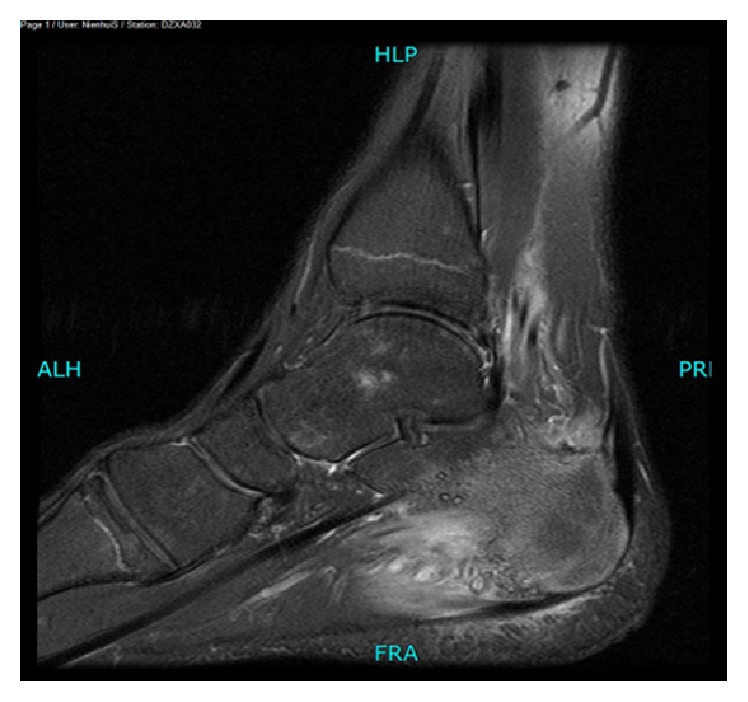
MRI T2 fat suppression: sagittal view of the left foot: high signal intensity of the soft tissue close to the plantar fascia. Secondary edema of bone marrow in the calcaneal bone.
